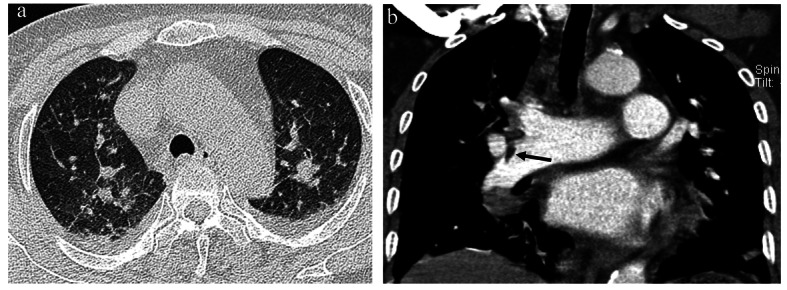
(a) A high resolution CT image of the lung reveals patchy ground glass opacities in both lung fields and bilateral pleural effusion. (b) A coronal reformatted image of the pulmonary artery shows a small filling defect in the right intermediate branch of the pulmonary artery and the mean attenuation value of the lesion is -42 Hounsfield units (HU), suggesting the presence of fat embolism (black arrow).
